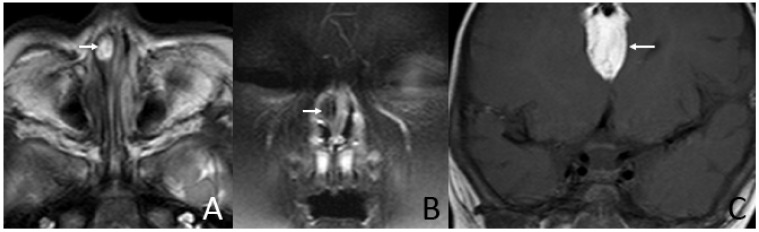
MRI of a 6-month-old and 18-day-old girl presenting shortly after birth with nasal obstruction. (A) Axial T2-weighted MRI shows a sausage-like mass (arrow) in the right nasal vestibule and nasal limen that is mainly high-signal with linear low-signal inside. (B) Coronal T2W-SPIR MRI shows the hyperintensity component of the mass suppressed (arrow). (C) Coronal T1-weighted MRI shows an irregular mass (arrow) containing fat in the intracranial midline that mainly has a high-signal with irregular linear low-signal inside.
